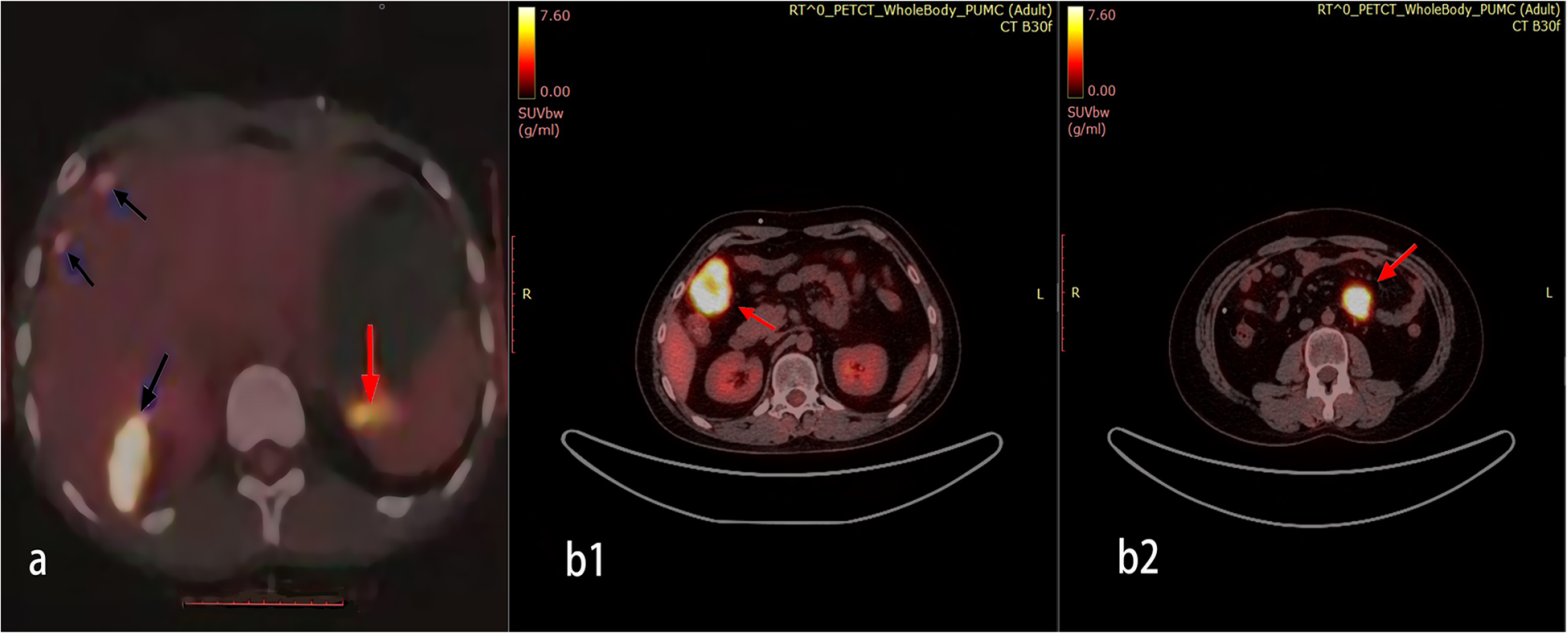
There were abnormal metabolic nodules near the inferior vena cava and the diaphragmatic apex, small nodules with slightly increased metabolism at the anterior right costal diaphragmatic angle, and extensive nodules with increased metabolism in the peritoneum (a) (marked in red and black arrows). Multiple-site SUV elevation in the abdominal cavity. The two main metabolic enhancement foci are concentrated in the right upper quadrant (b1, b2)
